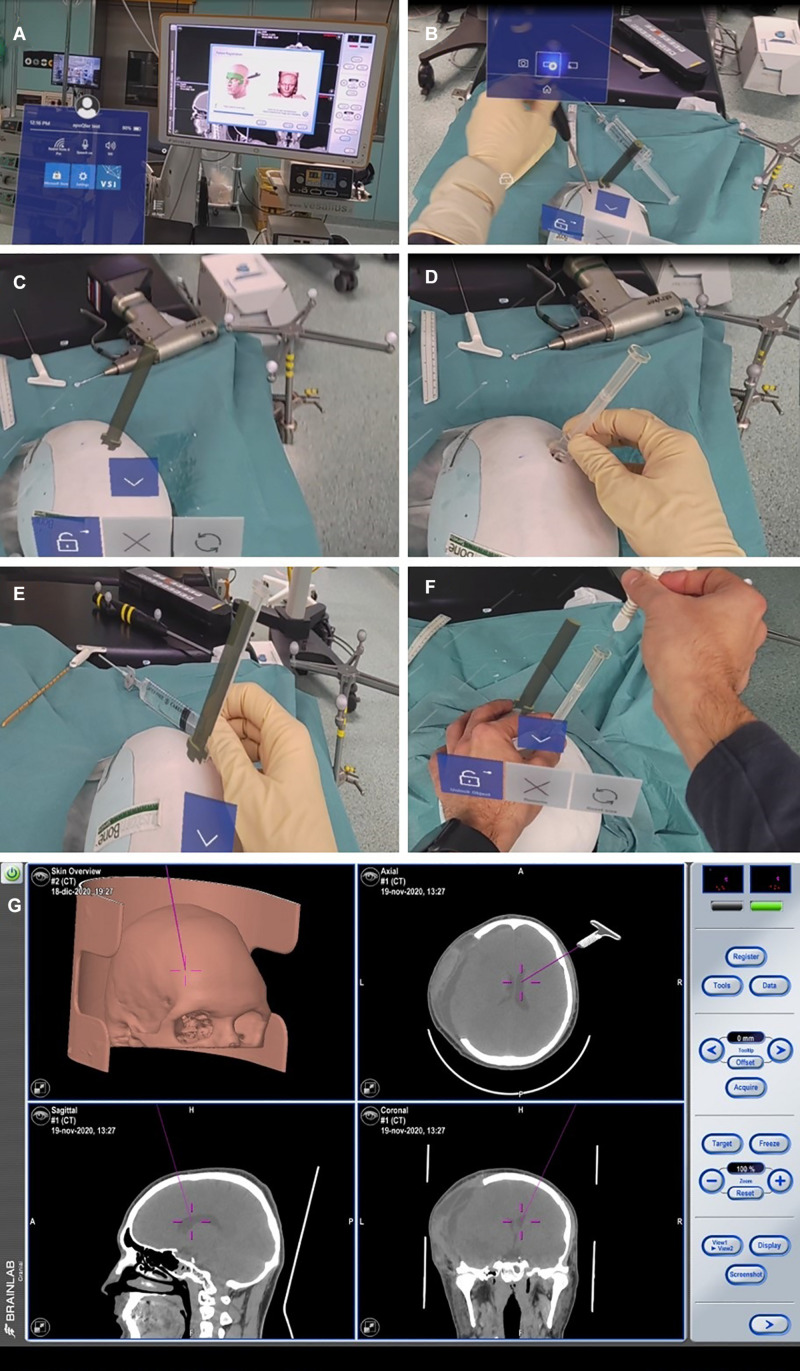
Finceramica plaster model navigation after merging the patient’s CT scan (already treated for post-traumatic craniotomy, but with no midline shift). (A,B) Superficial tracing for navigation registration (BrainLab) of the plaster model. (C) Entry point definition with a holographic representation of the device. (D) Positioning of the real device on the defined entry point. (E) Overlap of the holographic device with the real one, to match the entry point. (F) Introduction of the navigated stylet inside the device. (G) Its representation with the standard navigation.
